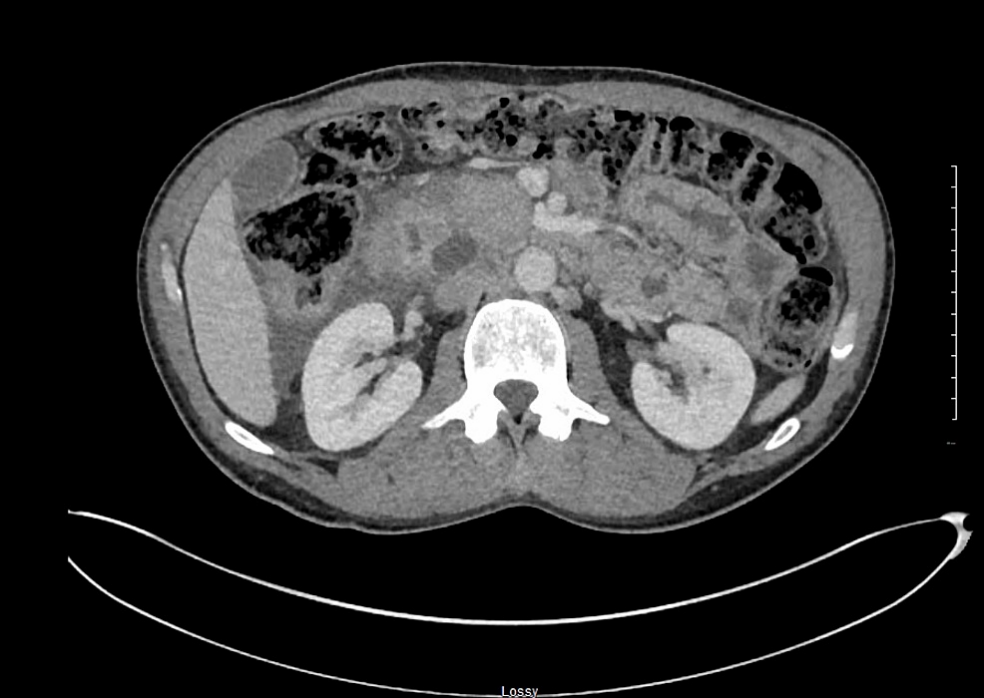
CT abdomen with contrast showed that there is s diffuse enlargement of the pancreas with the development of multiple pancreatic cysts along the pancreatic head and uncinate process measuring up to 2.4 cm
